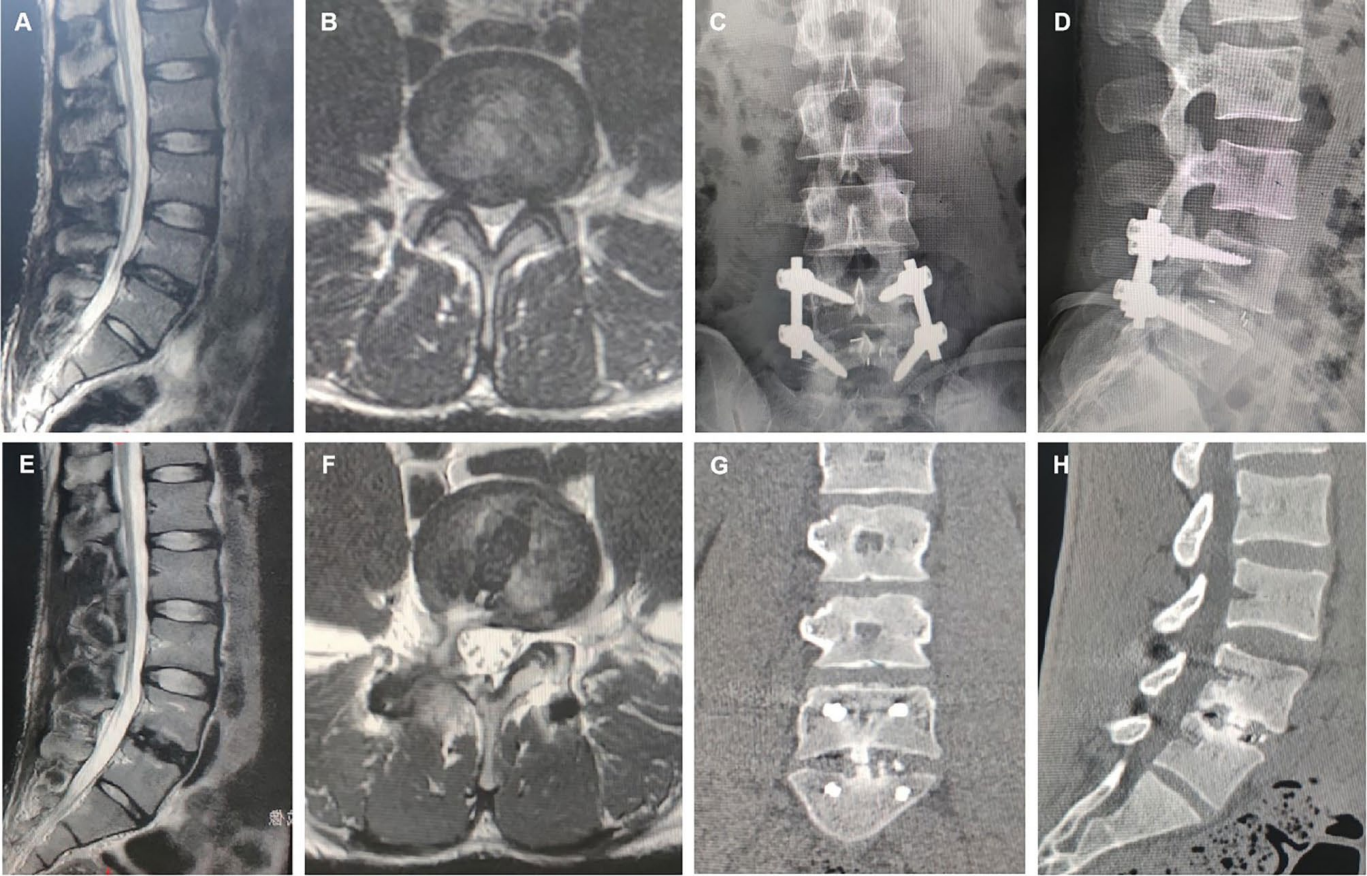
Male, 65 years old, L4/5 degenerative lumbar disc herniation. A. preoperative sagittal MR image; B. preoperative axial MR image; C. postoperative lateral X-ray image; D. postoperative anteroposterior X-ray image; E. postoperative sagittal MR image; F. postoperative axial MR image; G. 12-months postoperative coronal CT image; F. 12-months postoperative sagittal CT image
